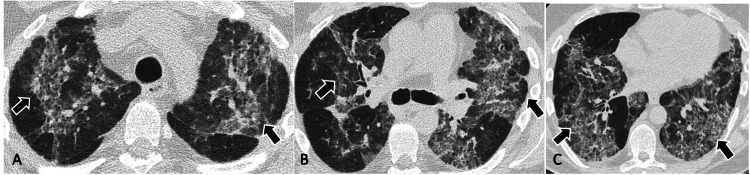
High-resolution CT performed two years after the initial scan. High-resolution axial CT at the upper lobes (A), carina (B), and lung bases (C) demonstrating ground glass opacities and findings of fibrosis with areas of traction bronchiectasis, overall encompassing more than two-thirds of the lungs (black arrows).
